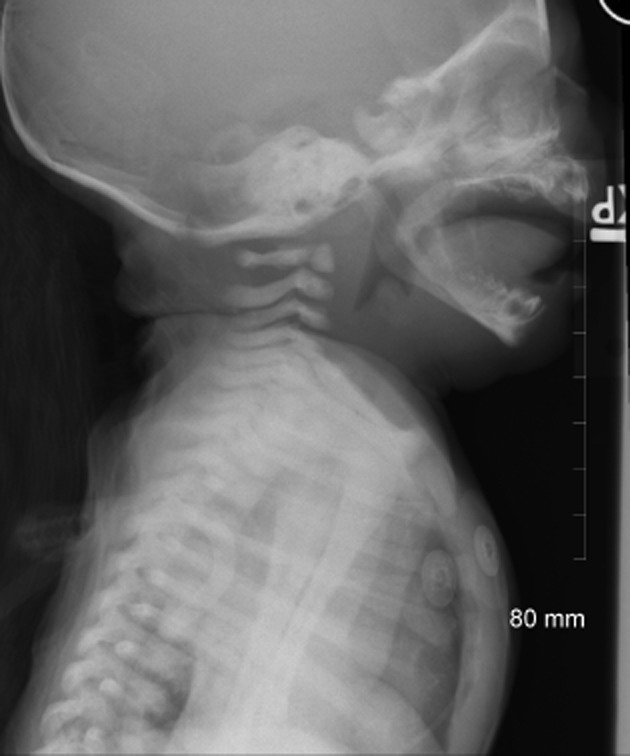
Soft tissue X-ray neck shows enlarged epiglottis and arytenoids, thick and convex aryepiglottic folds, and a distended hypopharynx consistent with epiglottitis (supraglottitis).
